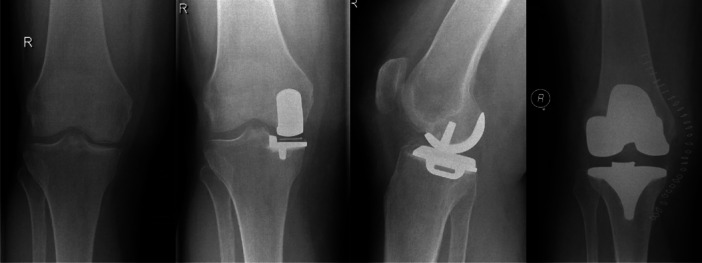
Example of a patient without bone‐on‐bone osteoarthritis on the preoperative radiograph. The post‐operative radiographs show a well‐aligned UKA. This patient was revised for unexplained pain. UKA, unicompartmental knee arthroplasty.
